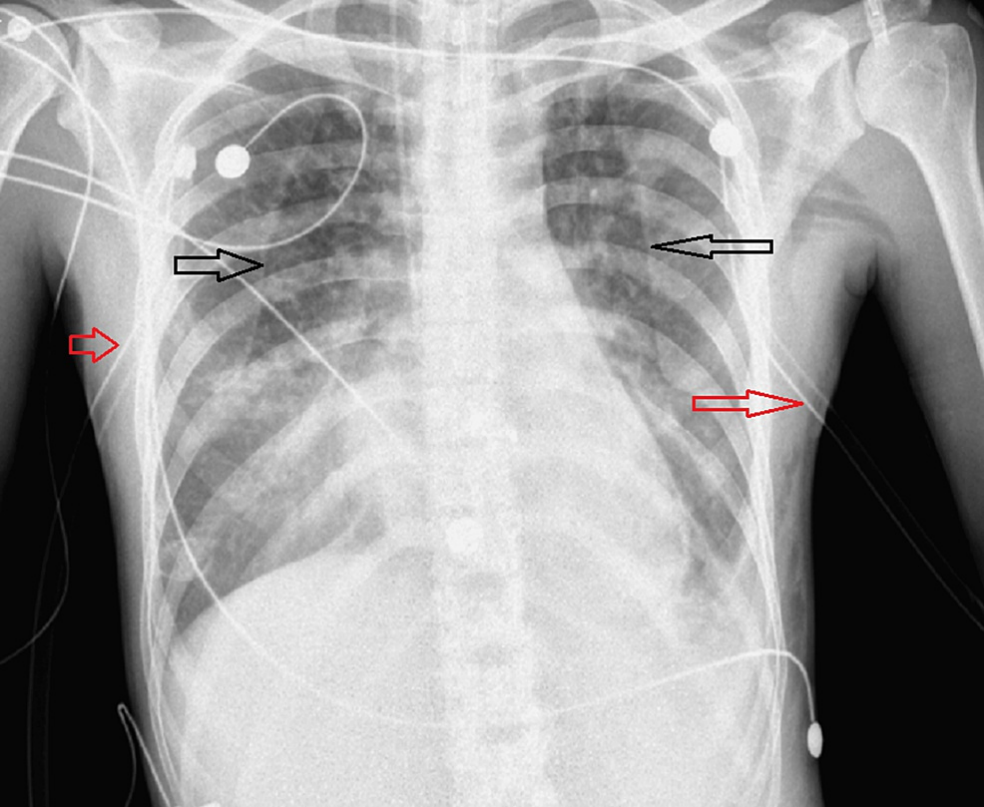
CXR with bilateral pneumothorax and chest tubes Black arrows point to the pneumothorax and red arrows point to the chest tubes. CXR: chest X-ray
